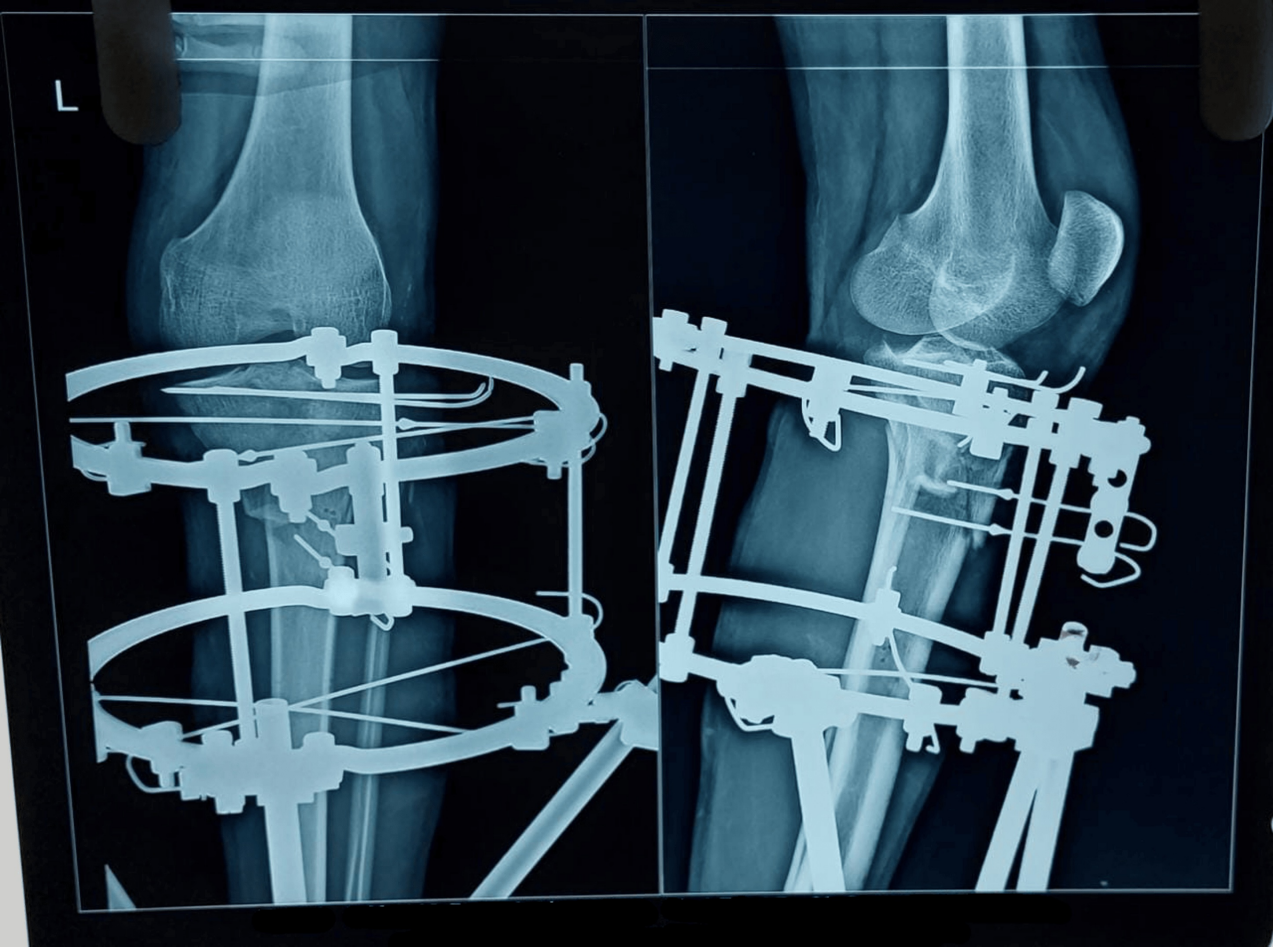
Postoperative X-ray after reduction and Ilizarov construct placement
